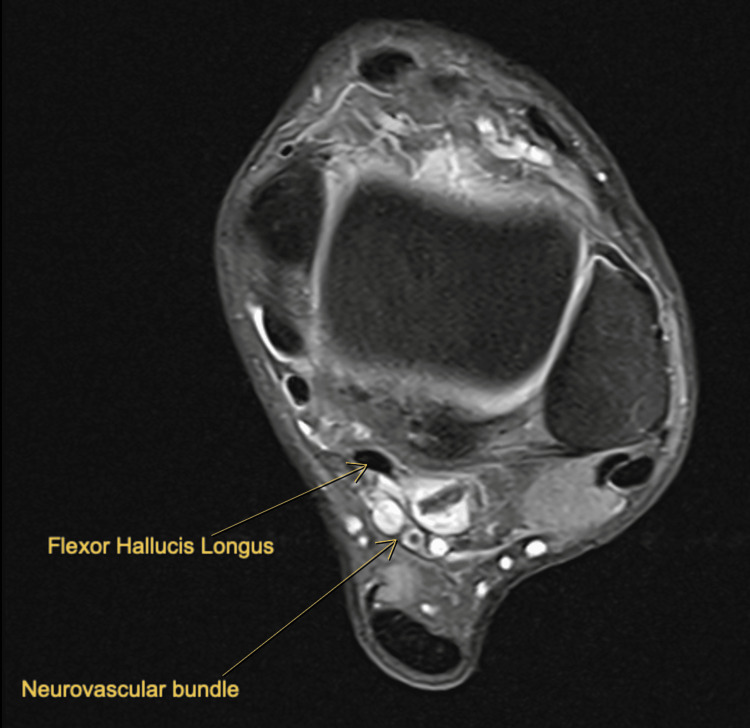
Axial fat-suppressed T2-weighted MRI of the left ankle Axial fat-suppressed T2-weighted MRI of the left ankle demonstrating the relationship between the flexor hallucis longus (FHL) tendon and the posterior tibial neurovascular bundle. The neurovascular bundle is located posterolateral to the FHL tendon, representing an atypical anatomical configuration.
